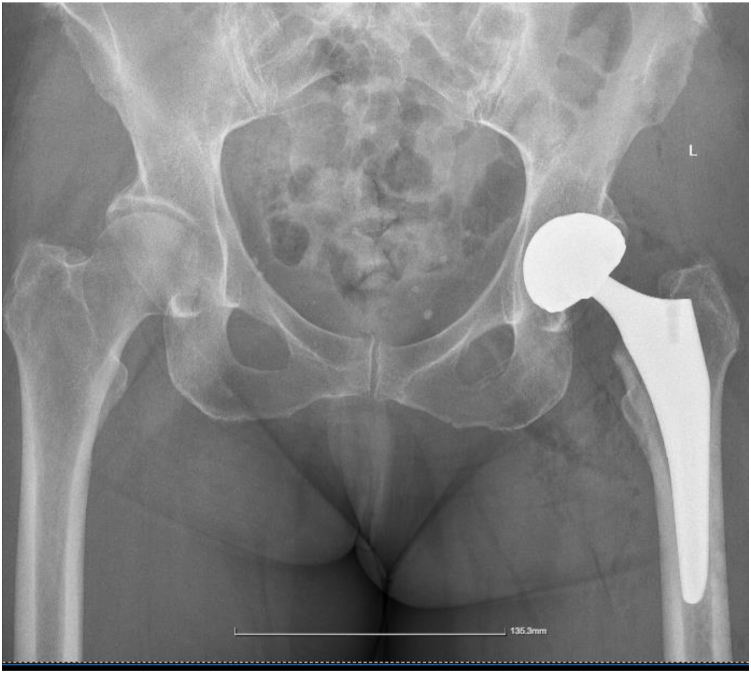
Postoperative anteroposterior X-ray view of the pelvis.
